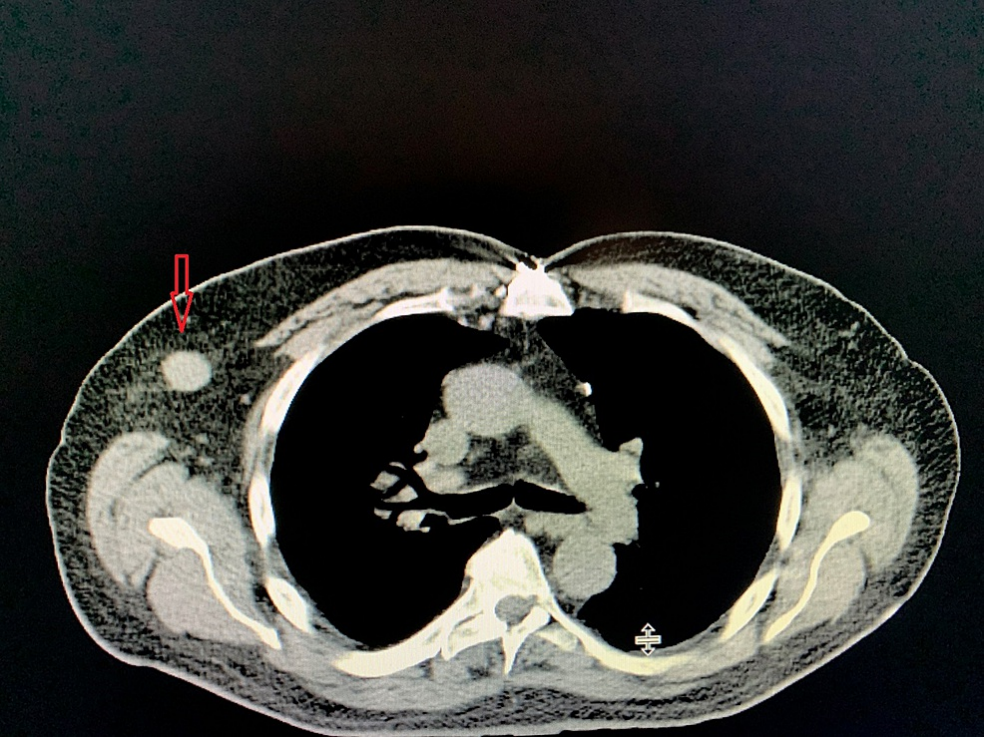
CT scan showing axillary mass/lymphadenopathy (red arrow)
